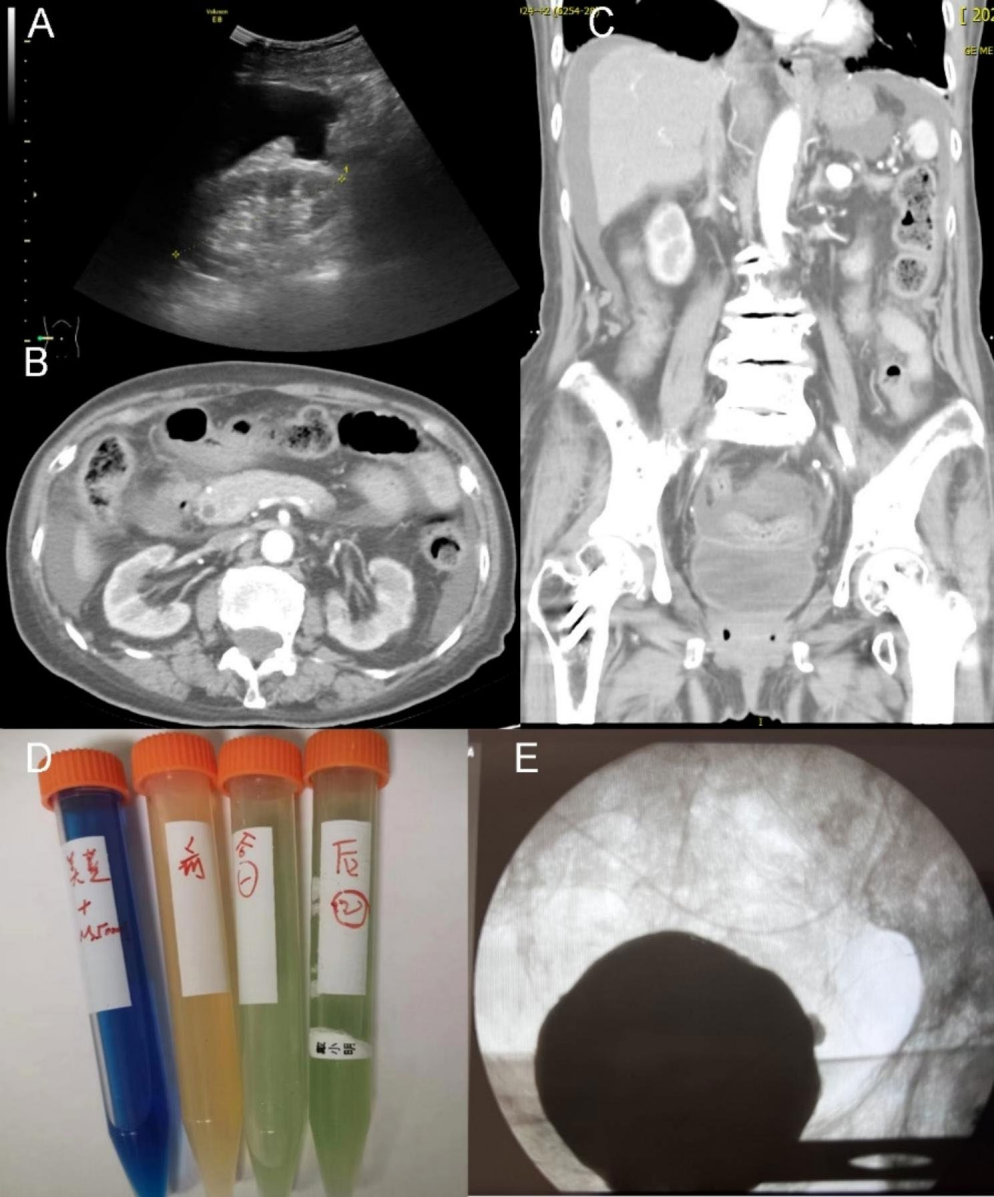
The main results for the examinations in the patient. Note: The kidney ultrasound revealed the presence of ascites and the absence of hydronephrosis in emergency room (A). Further contrasted computed tomography scan confirmed no hydronephrosis (B) and the presence of urine in the bladder (C). The methylene blue test conducted on the day of catheter placement yielded positive results (D). Arranged from left to right, the fluids exhibited the colors blue (methylene blue solution), yellow (ascitic fluid before the test), light green (ascitic fluid one hour after the test), and green (ascitic fluid two hours after the test), respectively. Subsequent to three days of indwelling Foley catheterization, a contrast-enhanced fluid containing a radiopaque agent was infused into the bladder via the catheter, and cystography examination revealed no evidence of leakage (E)
